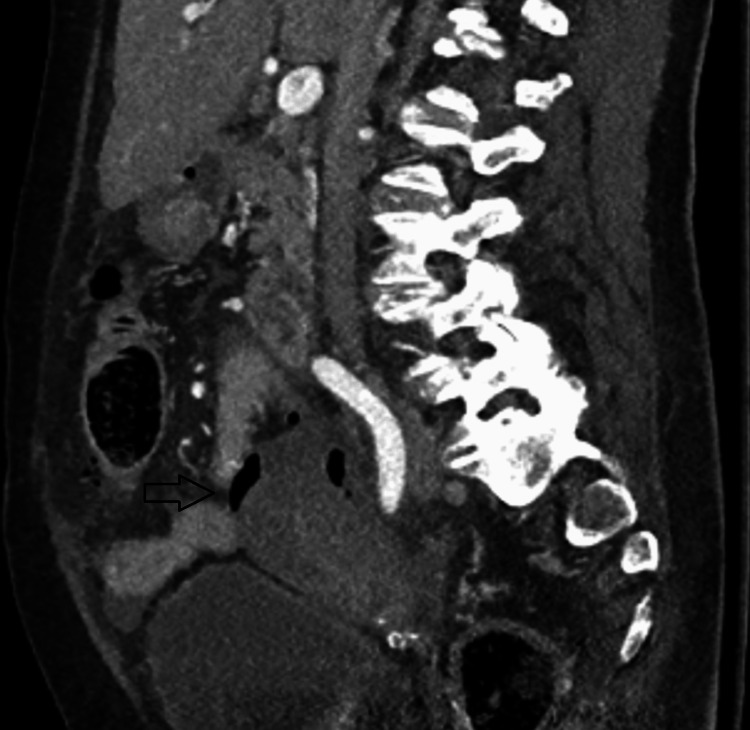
Sagittal cut from CT abdomen/pelvis showing periuterine free air
